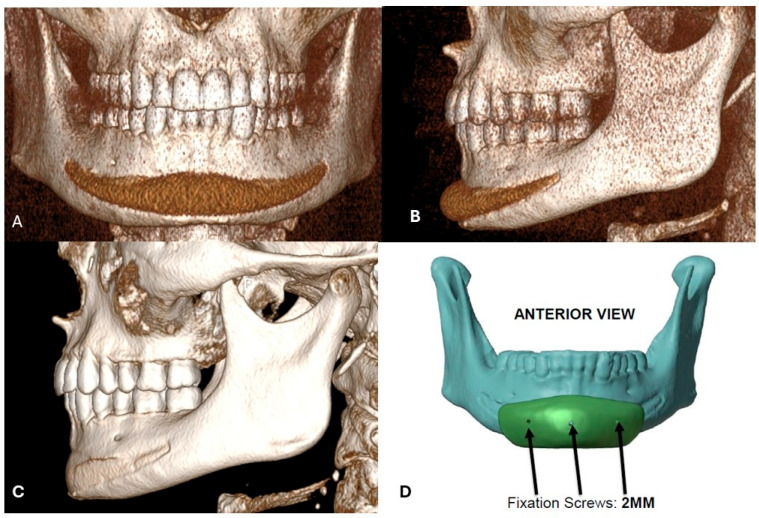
A 30-year-old Caucasian man who presented for consultation after a failed chin implant placed to enhance the lower third’s masculine features. A stock silicone implant had been used and did not provide the expected volumizing effect or masculinization of the mandible (A). CT imaging revealed the implant caused bone resorption along its entire length- anterior view and (B) lateral view. (D). After the digital removal of the silicone implant, reconstructed images confirmed resorption of the bone underneath the implant (C). The surgical plan called for the removal of the silicone implant and immediate placement of a custom-made PEEK chin implant to augment the anterior lower third and create a squarer, more masculine appearance (D).
